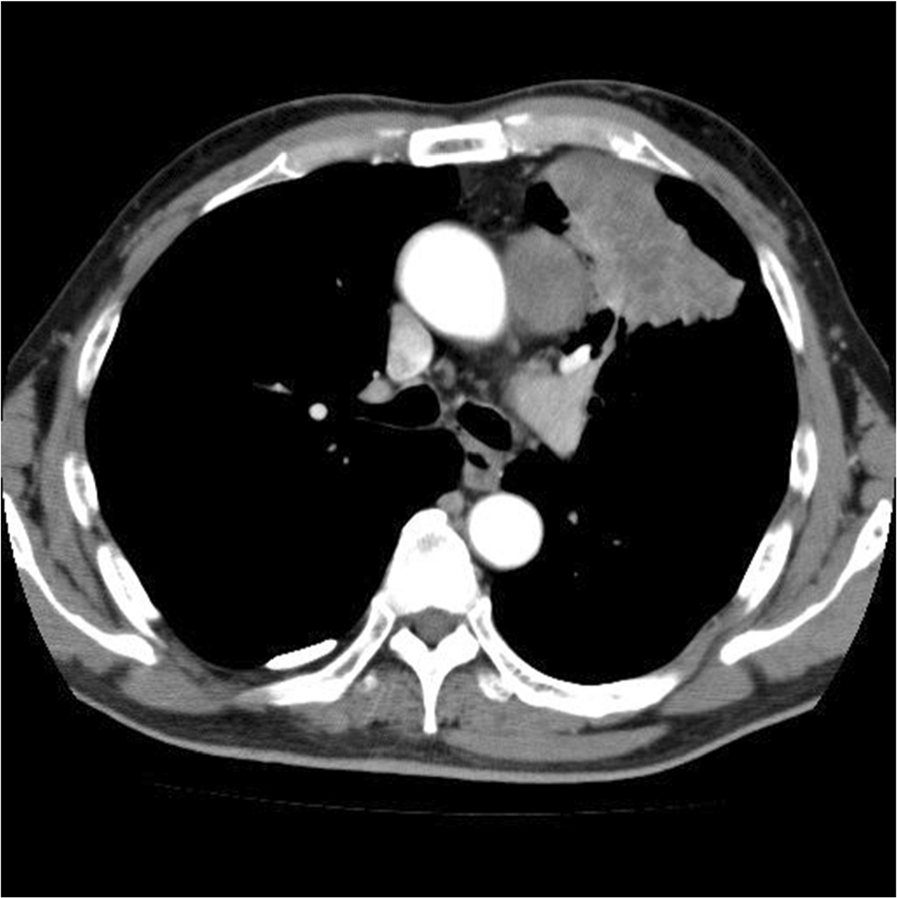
Enhanced computed tomography of the chest showed a 60-mm distal aortic arch aneurysm and a 60-mm mass shadow in the left upper lobe. The aortic arch aneurysm was connected to the pulmonary mass in the left upper lobe
